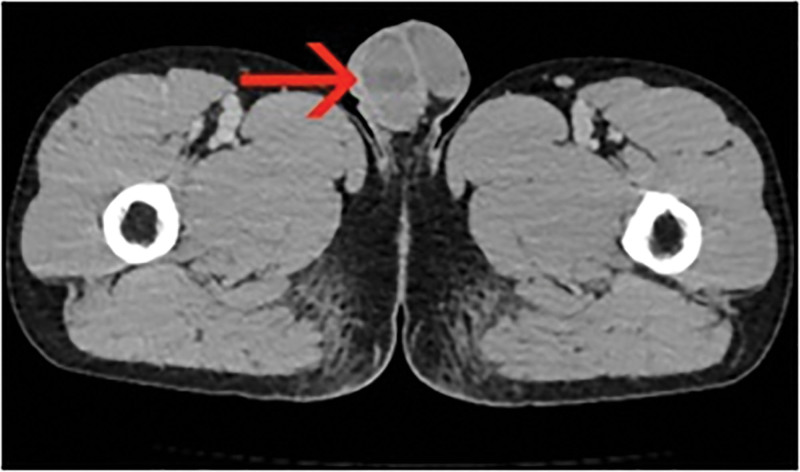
CT shows hypoechoic non-enhanced area in the right testis. CT = computed tomography.
